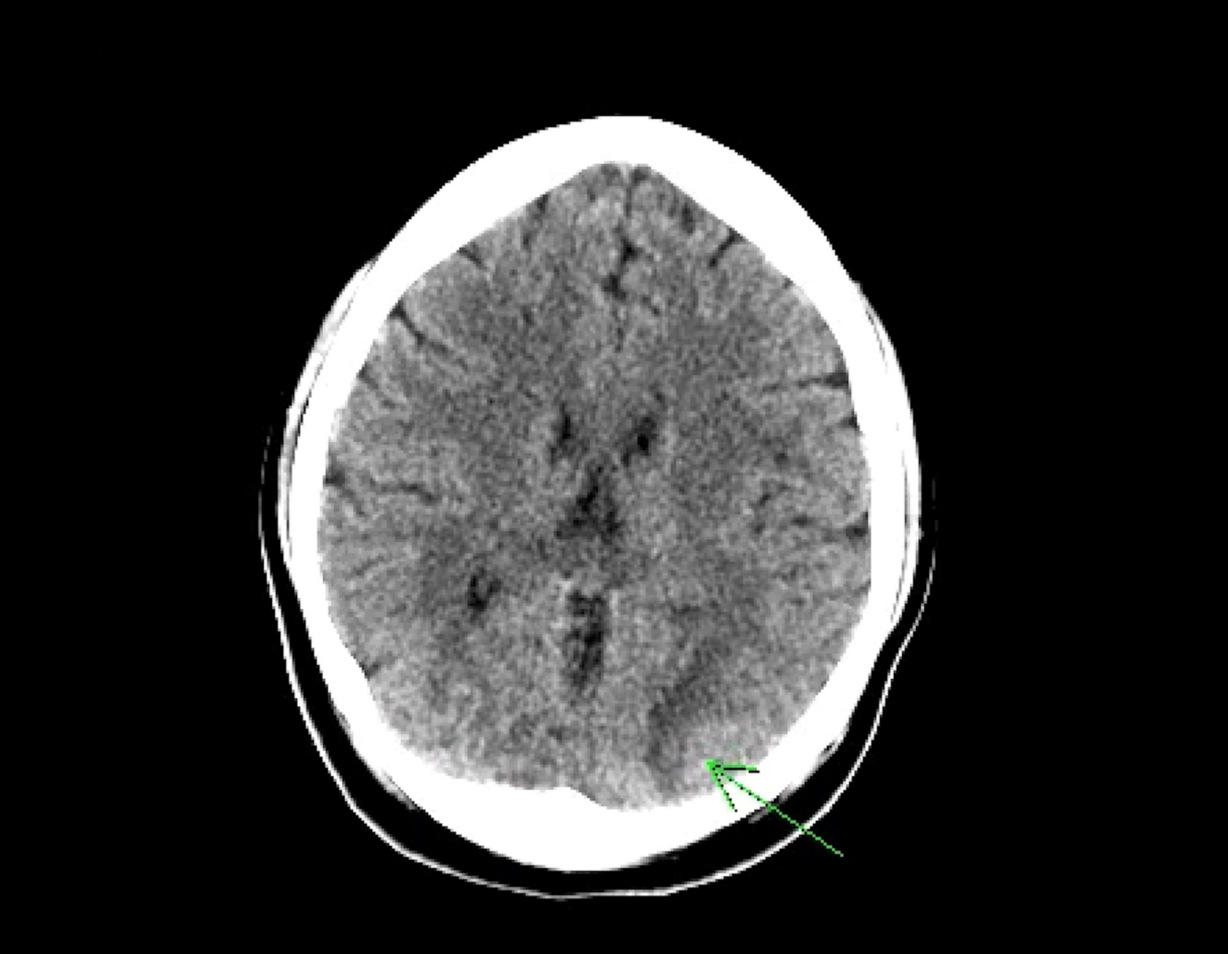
CT Head Without Contrast The left occipital lobe has low density noted with loss of gray-white differentiation concerning for possible acute infarction
